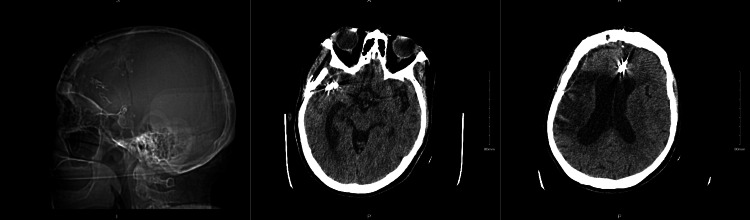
Postoperative CT scan demonstrating placement of vascular clips at the large thrombosed right MCA bifurcation aneurysm and at ACA aneurysms ACA: anterior cerebral artery; MCA: middle cerebral artery
